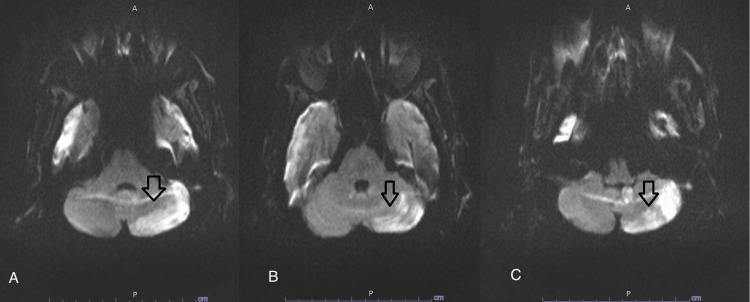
MRI diffusion trace sequence of the cerebellum Magnetic resonance imaging (MRI) brain diffusion trace sequence showing evidence of diffusion restriction affecting the left cerebella ray hemisphere (arrows in A, B, and C)
